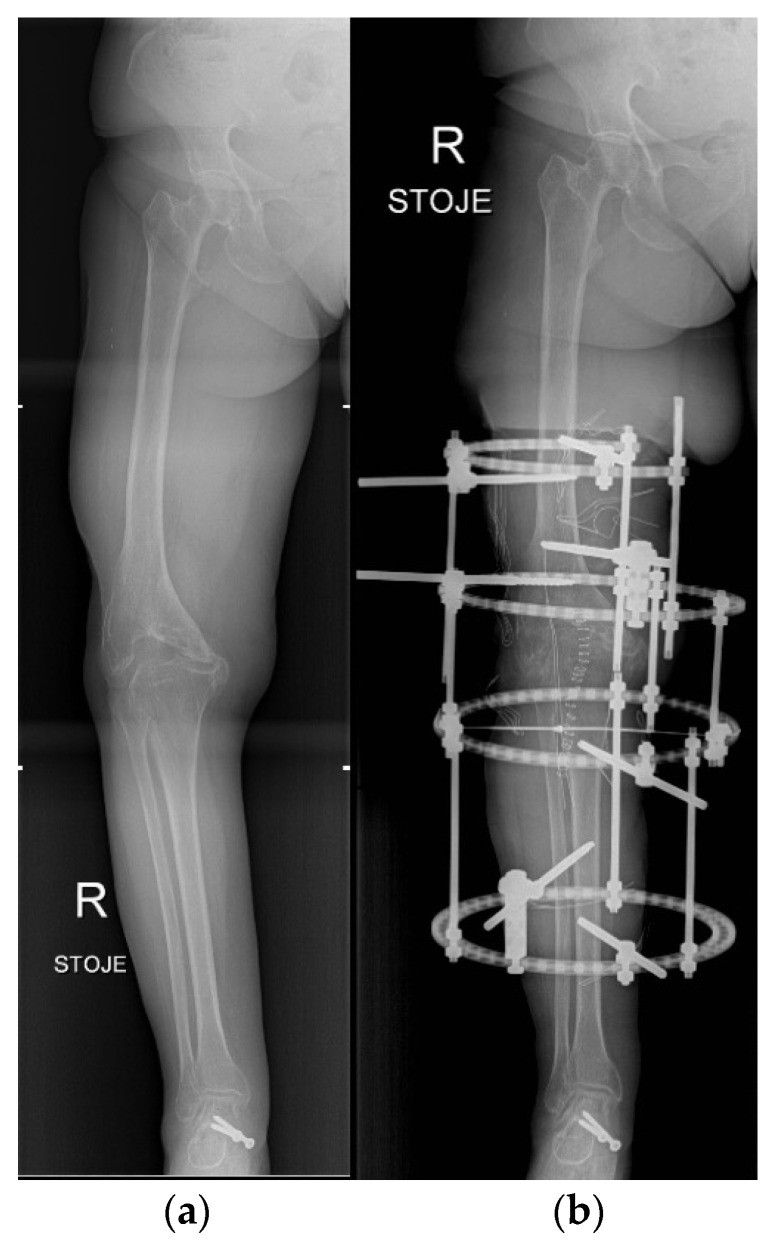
Knee arthrodesis. (a) preoperative X-ray of residual knee deformity after severe trauma; (b) postoperative X-ray after limb salvage knee arthrodesis with Ilizarov technique.
